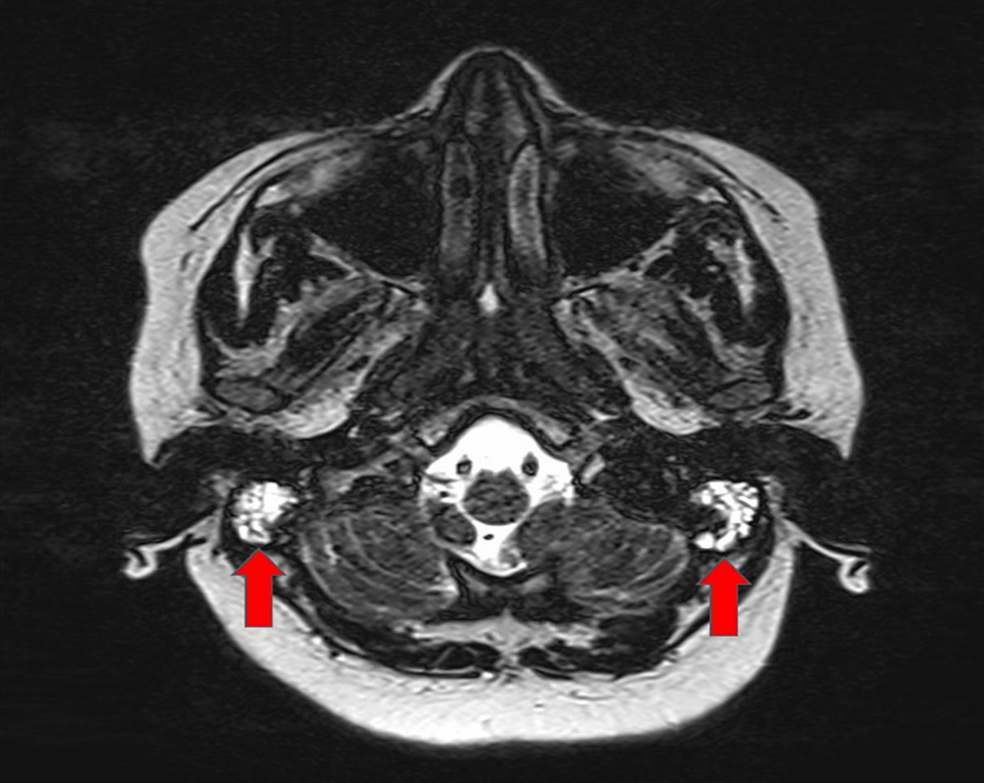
Fluid presence in mastoid cells on preoperative short-term T2 sequence MRI MRI: magnetic resonance imaging
